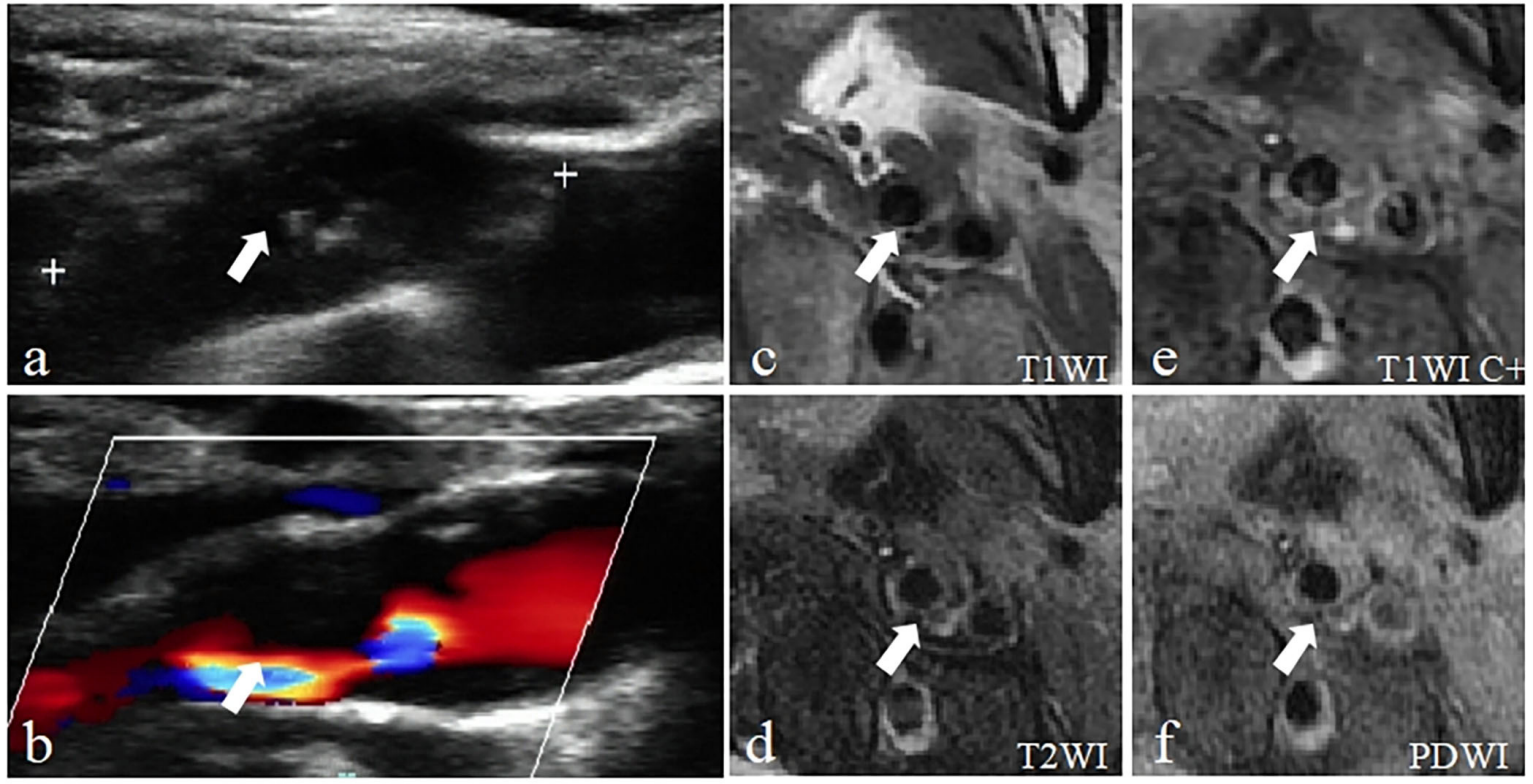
Example of a vulnerable carotid plaque of a patient using Carotid ultrasound (CDU) and High-resolution magnetic resonance imaging (HRMRI). (a) Gray-scale longitudinal section scan shows heterogeneous plaques in the posterolateral wall of the left carotid bulb extended to the internal carotid artery with a large hypoechoic area inside (arrow). (b) CDFI longitudinal section scan showed that blood flow signals were visible in the residual lumen (arrow). (c) The plaque is characterized by the isointensity on T1-weighted imaging (T1WI) (arrow). (d) T2-weighted imaging (T2WI) showing slightly high signal intensity (arrow). (e) Enhanced T1-weighted imaging (T1WI C+) showing regional enhancement within the AP (arrow), defined as neovascularization or inflammation, indicate the plaque is more likely to be vulnerable. (f) Proton density-weighted imaging (PDWI) showing slightly high signal intensity within the AP (arrow).
